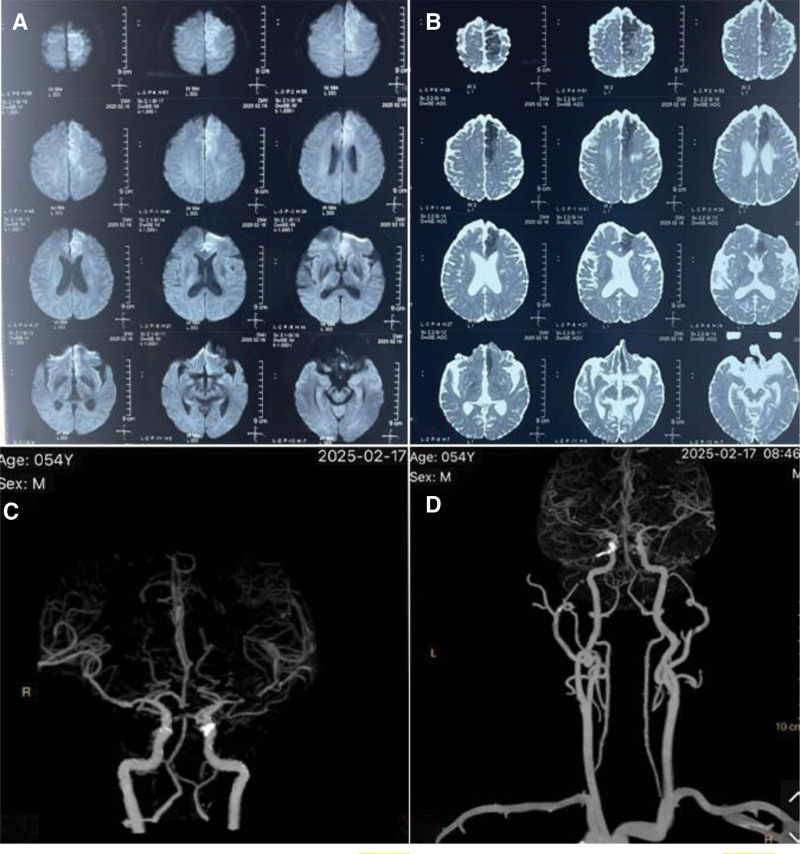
(A) Diffusion-weighted imaging. (B) Apparent diffusion coefficient image. (C) CTA of the head. (D) CTA of the head and neck. CTA = computed tomography angiography.
